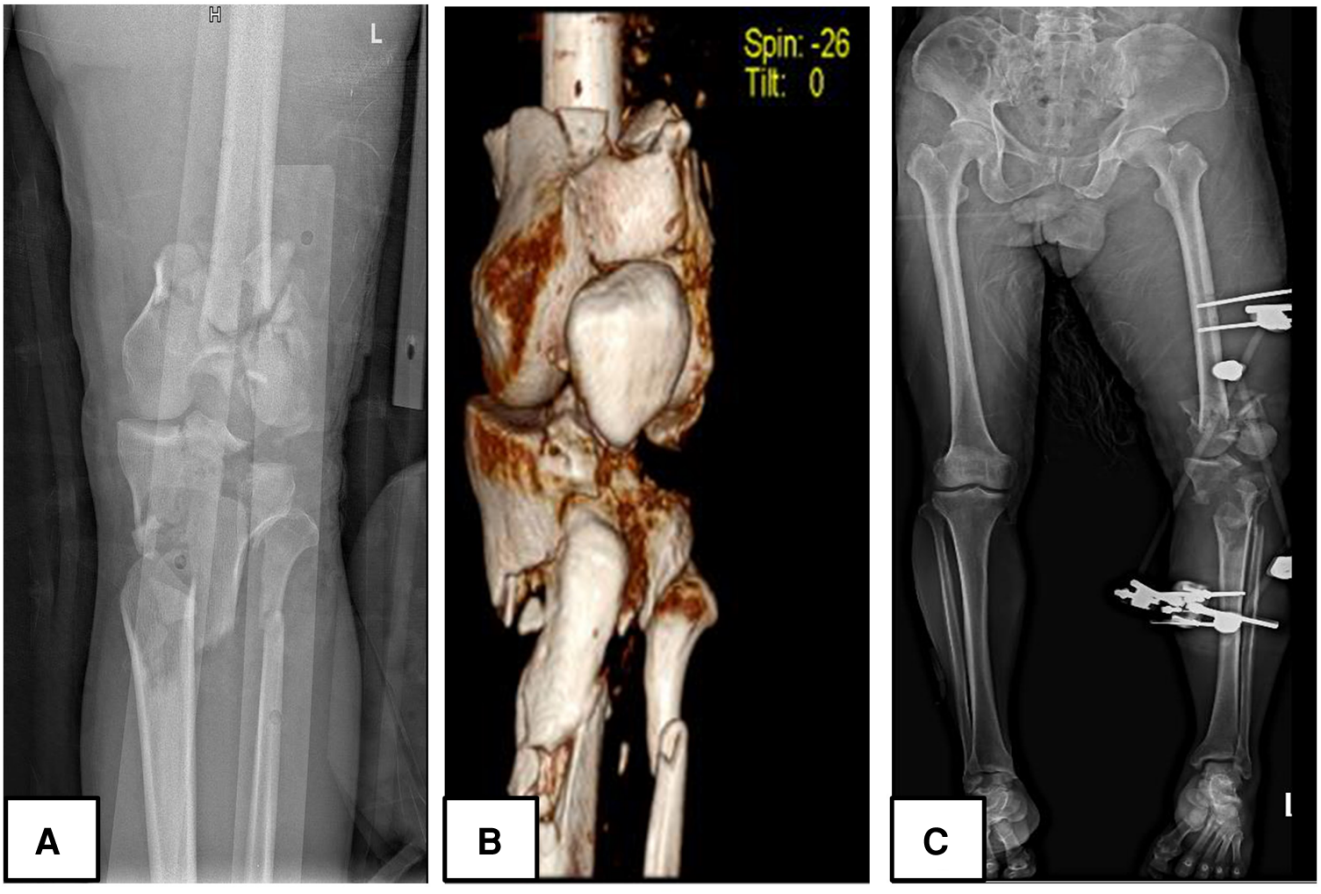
A 65 years-old male patient (patient number 34) who sustained a Fraser type IIC floating knee (left limb) following a road traffic accident managed surgically; (A,B) demonstrated preoperative plain radiograph and 3D reconstruction CT images respectively; (C) displays a postoperative plain radiograph of the lower limb, the femur and tibia were managed with knee spanning external fixation.
